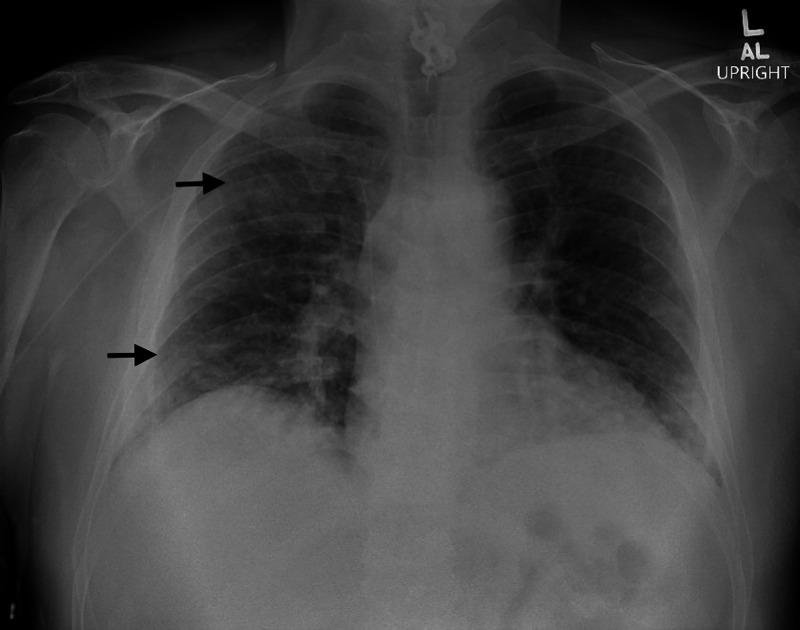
Portable CXR demonstrating multiple opacities concentrated in the right upper and lower lobes (black arrows). CXR, chest X-ray
